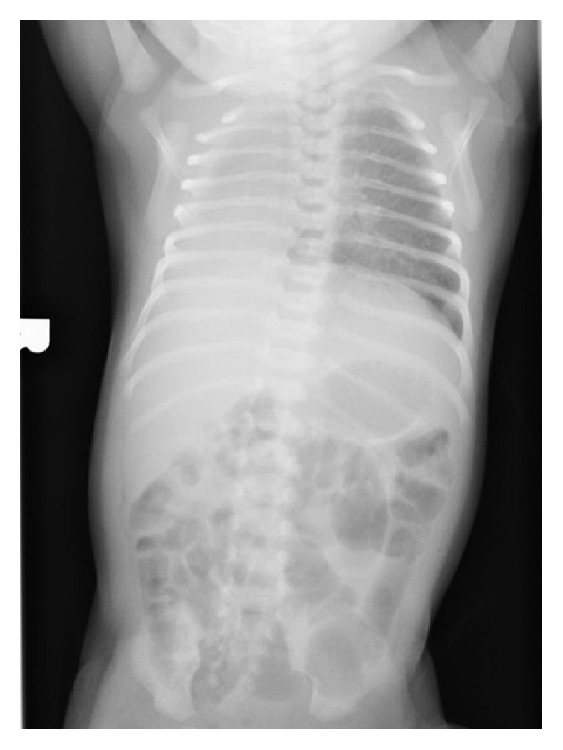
Chest X-ray revealing right lung agenesis and dextrocardia.
